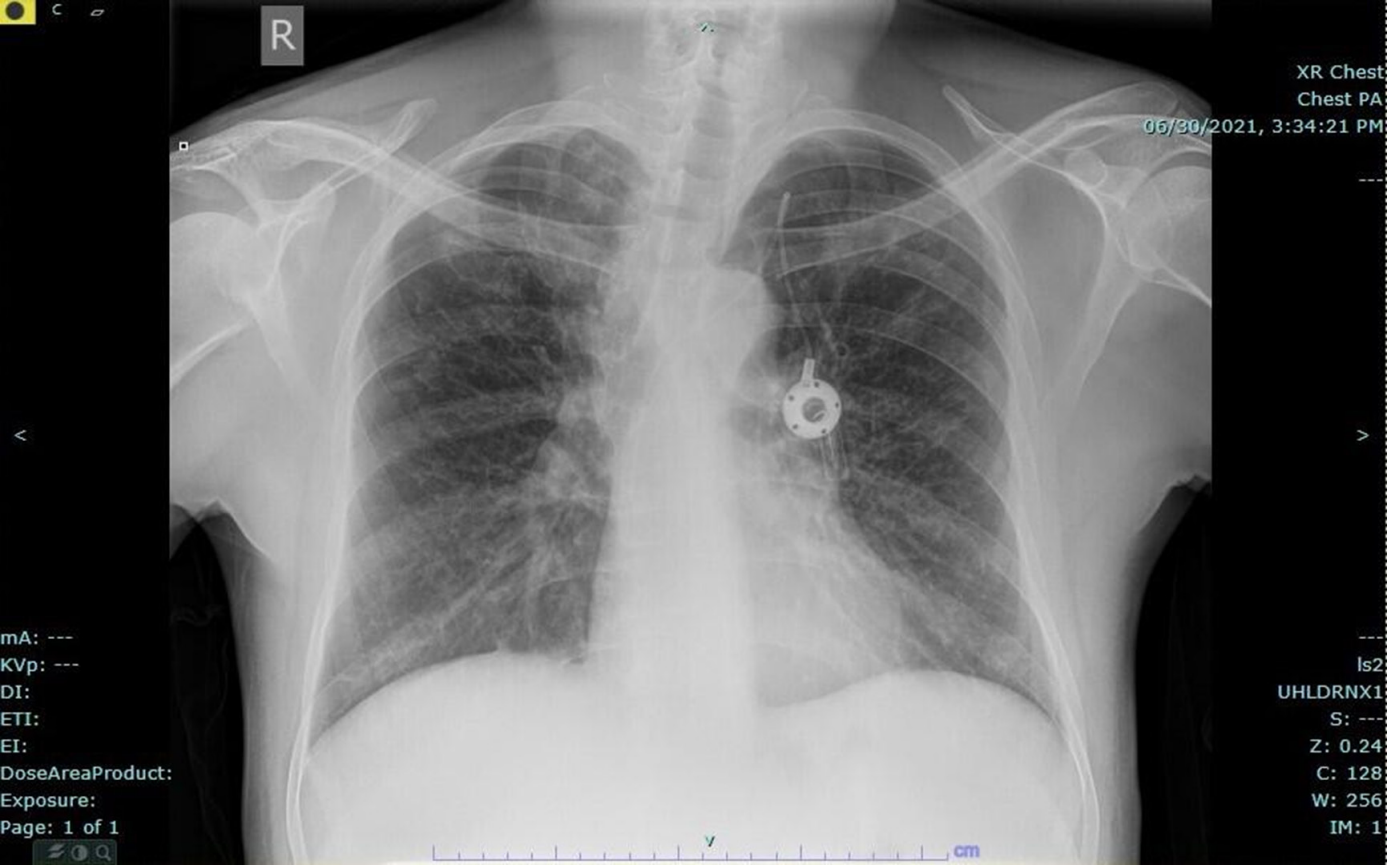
Chest x-ray of our patient at admission with respiratory exacerbation in July 2021.
